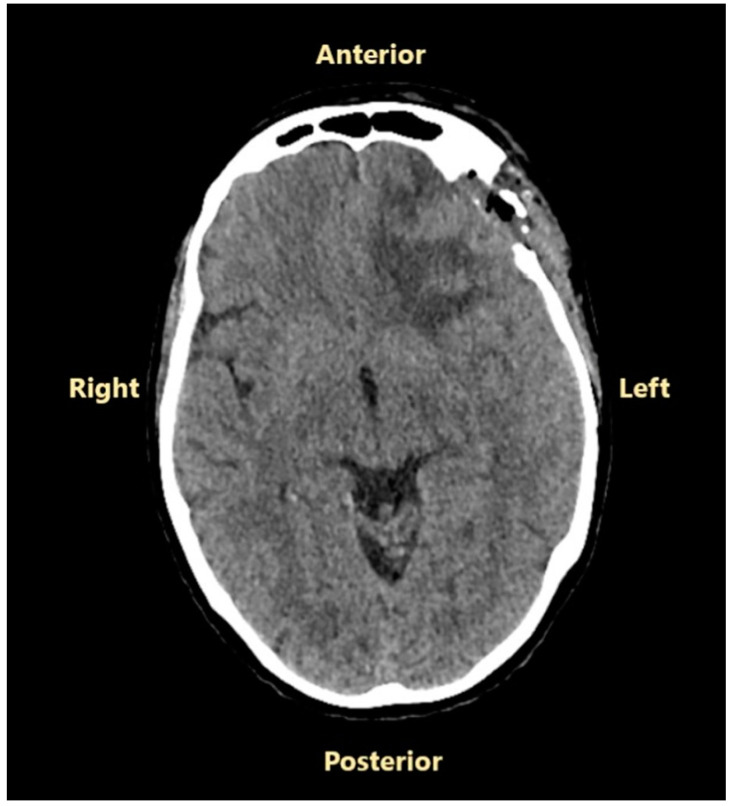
Postoperative native cranial CT scan, performed in May 2024.
